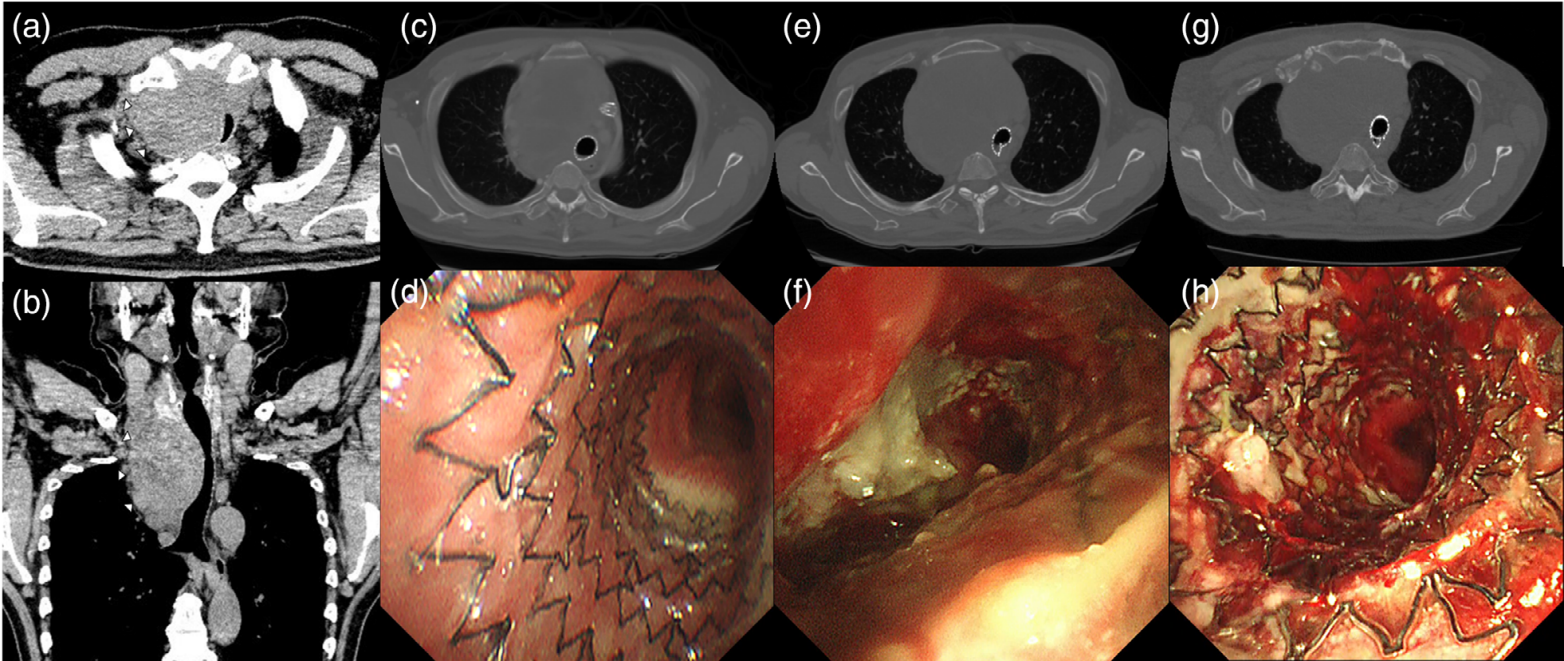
Computed tomography (CT) images of tracheal stenosis with severe extrinsic compression pattern (arrows) in cross‐sectional image (a), and in multiplanar reconstruction image (b). CT and bronchoscopic findings of the AERO stent distal portion; CT finding after first stent placement (c), bronchoscopic finding after first stent placement (d), CT finding at stent collapse by tumor growth (e), bronchoscopic finding at stent collapse by tumor growth (f), CT finding after additional stent placement with stent‐in‐stent method (g), bronchoscopic finding after additional stent placement with stent‐in‐stent method (h).
